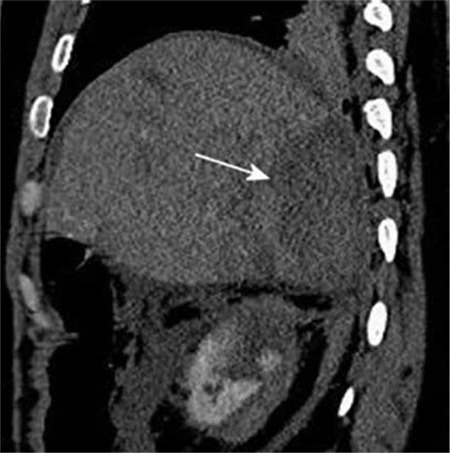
Contrast-enhanced computed tomography sagittal scan reconstruction showing subcapsular haematoma in the right liver lobe (arrow).
